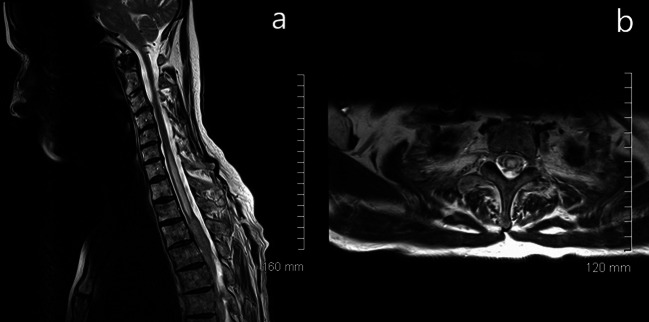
Sagittal T2-weighted MR image (a) demonstrates a hyperintense cord lesion extending from C6 to T2/T3. Axial image (b) confirms a central location within the cord
